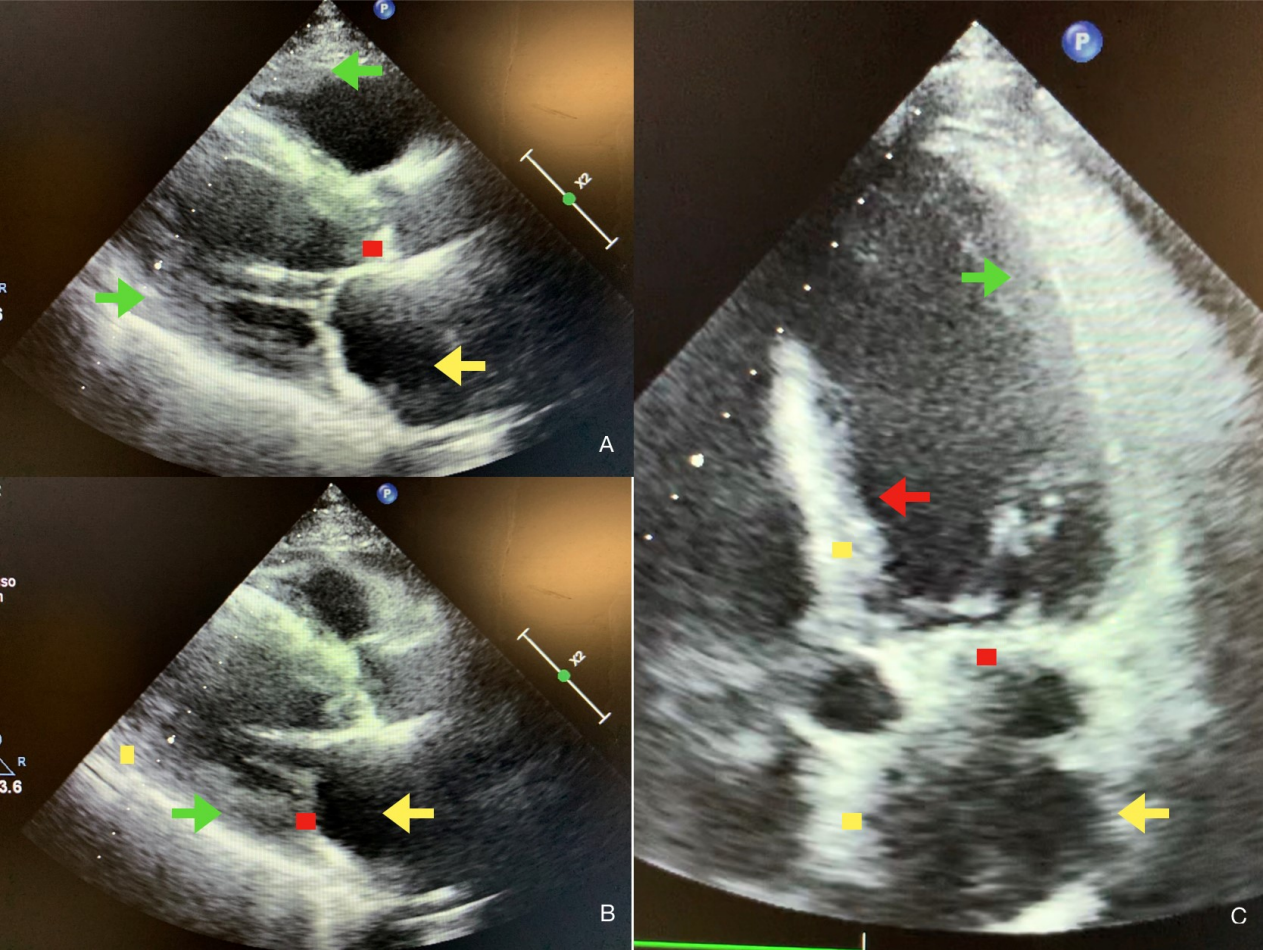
Echocardiographic features of atrial amyloidosis. Parasternal
